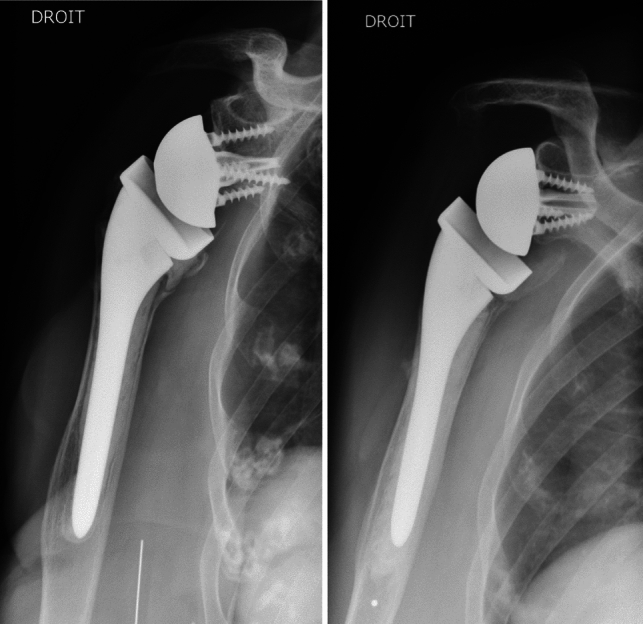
Postoperative radiographs: tuberosities reinserted (left image) or not reinserted (right image)
